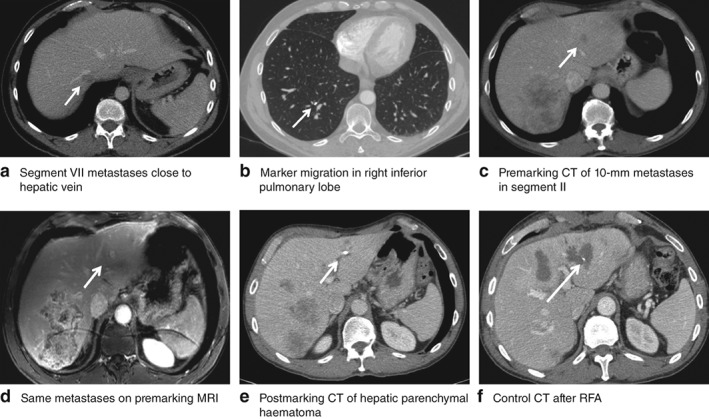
Fiducial marker placement‐related complications. a,b Fiducial marker migration: a premarking CT scan with metastases of segment VII close to the hepatic vein; b postmarking CT scan with fiducial marker migration in the subsegmental branch of the posterobasal segment from the inferior right lobe with no evidence of pulmonary embolism. c–f Hepatic parenchymal haematoma: c premarking CT and d premarking MRI scans showing a 10‐mm metastasis in segment III (white arrow); e control CT scan the day after marking showing a hepatic parenchymal haematoma; f control CT scan after radiofrequency ablation (RFA)
